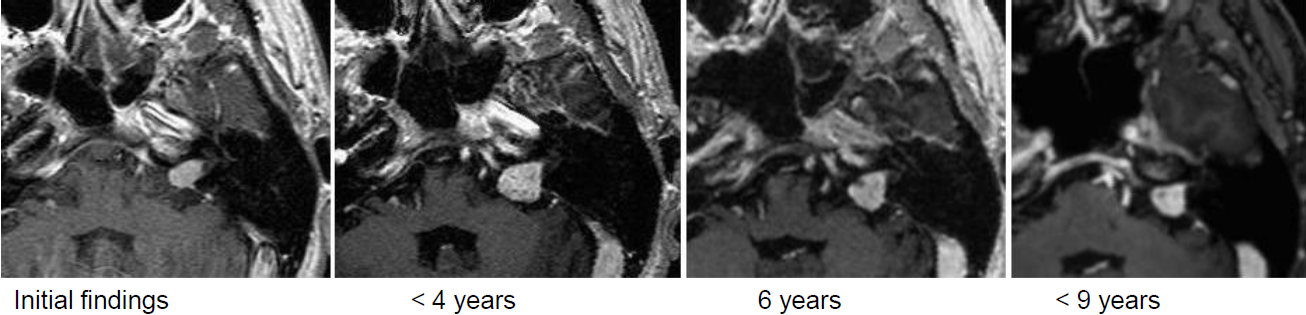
In the first years, the MRI follow-up control (T1-w + CM + contrast agent application) show a growing tumor with nearly unchanged contrast enhancement, only after 6 years, the tumor volume decreases.
